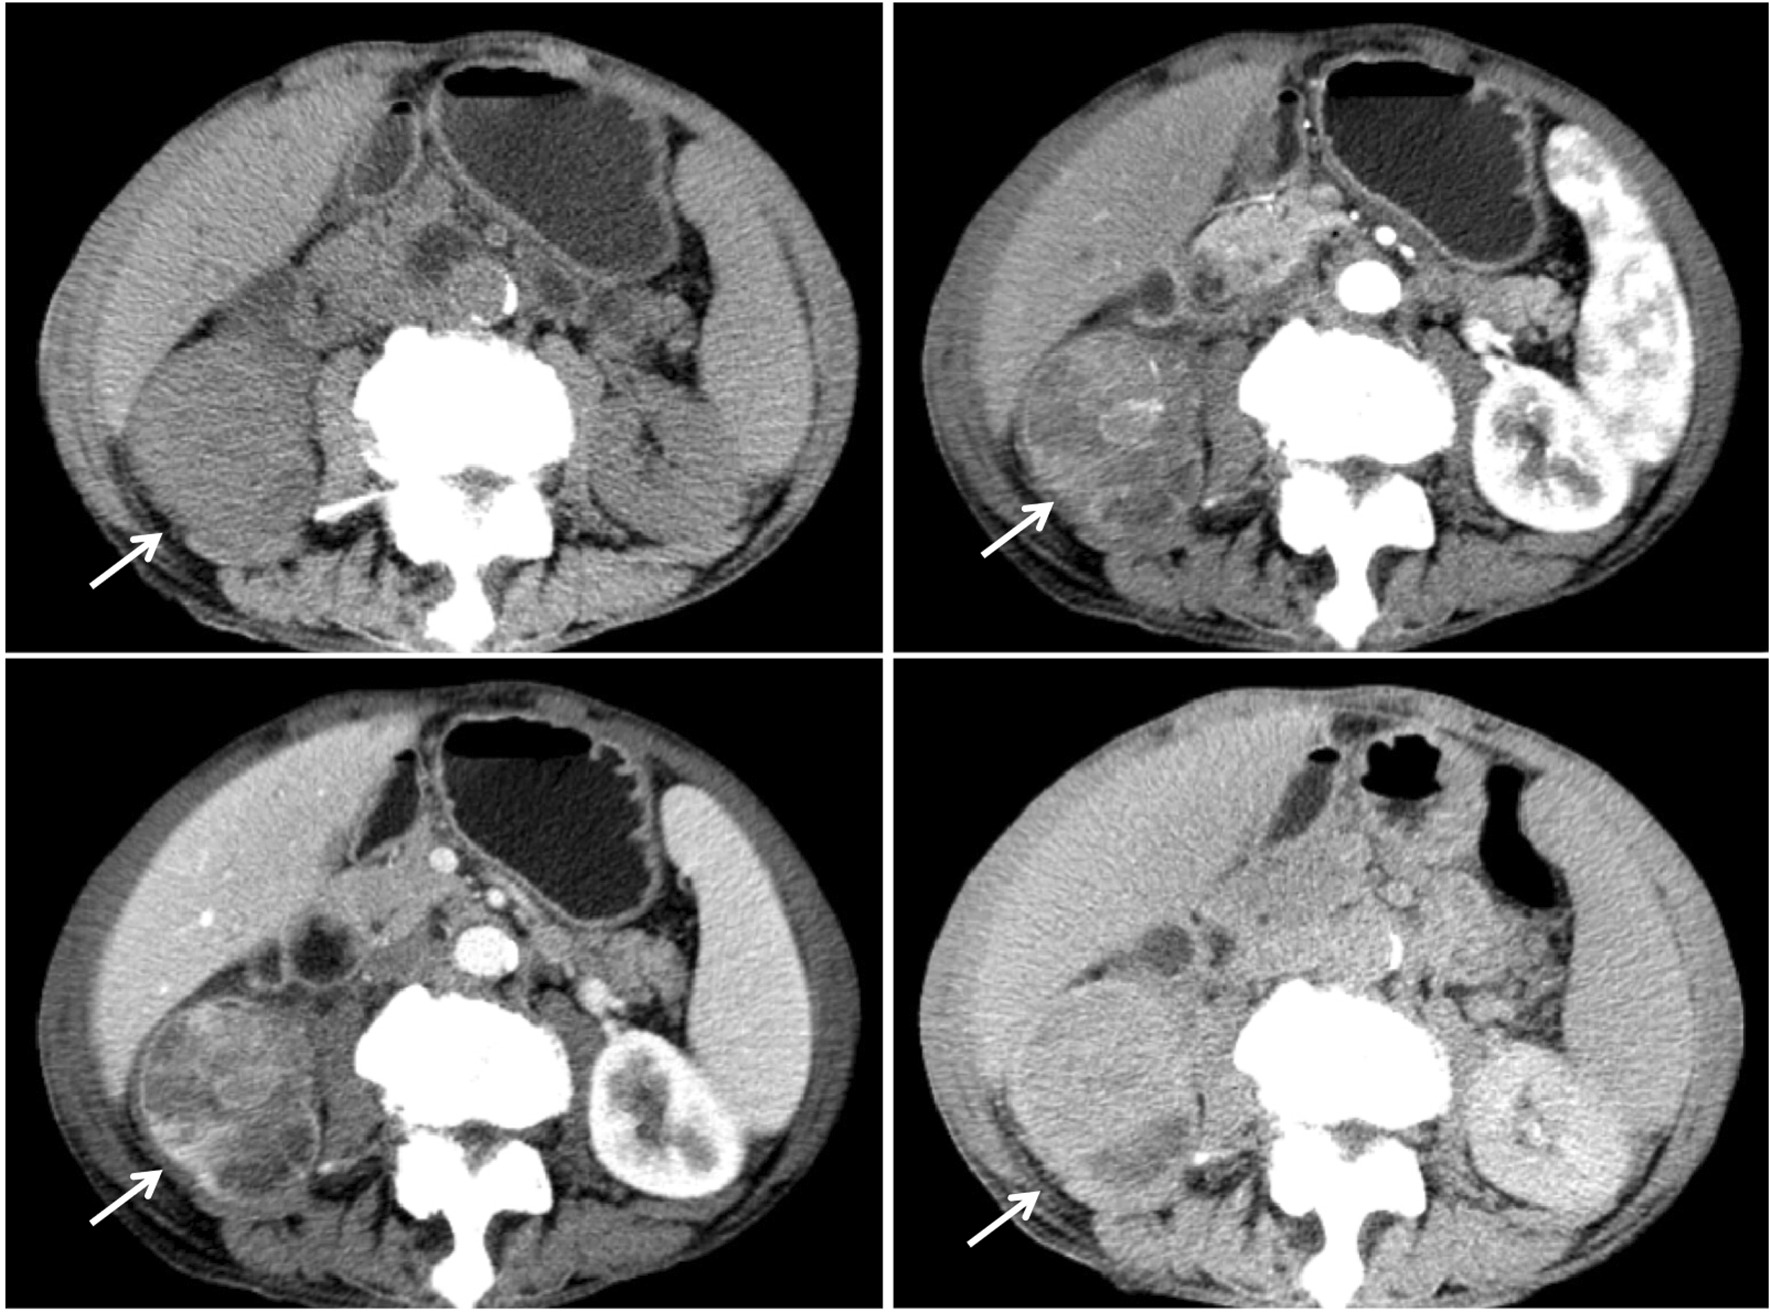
Case 5: axial unenhanced/enhanced CT image of the CDC shows that the right renal medulla is unclear. The internal density is uneven with light-moderate delayed enhancement (arrows)
